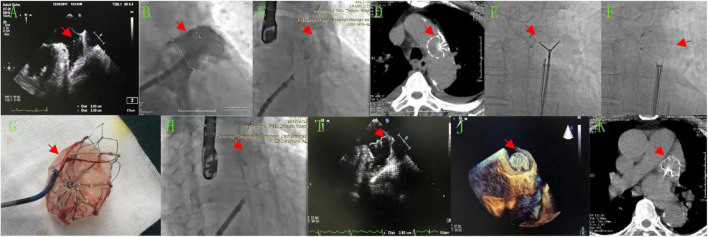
After LAAC, the occluder fell off into the aortic arch and was feathered out, before the 33-mm Watchman device was occluded again. (A,I,J) LA TEE images. (A) Preoperative LAA: ostial of 25–30 mm and an effective depth of 28 mm. (I,J) Two-dimensional and 3-dimensional images after the second closure with the Watchman device. (B,C,E,F,H) DSA images. (B) Preoperative LAA with an opening of 28 mm and a depth of 31 mm. (C) After occlusion with the 33-mm Watchman device. (E,F) Endoscopic Raptor forceps were used to clamp the umbrella trabeculae and drag it into the sheath. (H) Second closure with the Watchman device. (D,K) Chest CT image. (D) Occluder in the aortic arch. (K) LAAC with the Watchman device. (G) Modified occluder.
